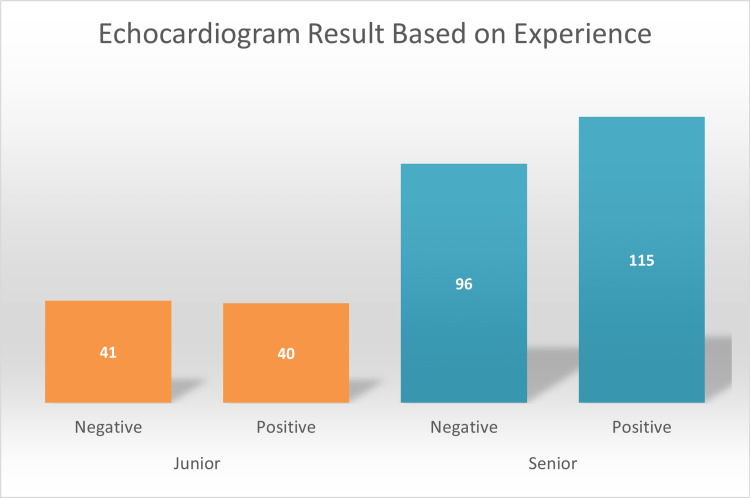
Echocardiogram results based on experience.
